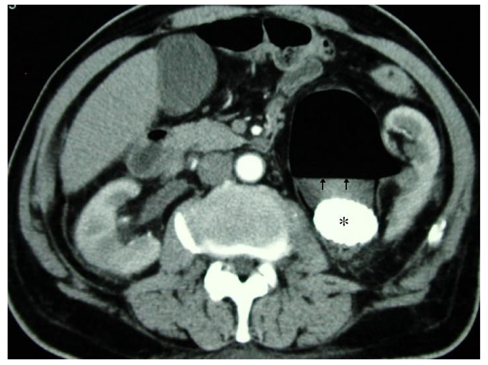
Axial image of contrasted CT scan of the abdomen showing a large obstructing calculus (*) at the left pelvi-ureteric junction, causing gross left hydronephrosis with gas (arrow) noted within the left pelvicalyceal system.
